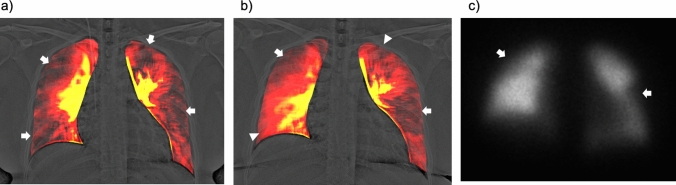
Dynamic chest radiography (DCR)-based detection of acute pulmonary thromboembolism (PTE) progression to chronic thromboembolic pulmonary hypertension (CTEPH) in a 32-year-old woman. In the acute PTE phase, DCR demonstrated multiple perfusion defects, suggesting pulmonary embolism in both lungs (a, arrows). Even after 6 months of anticoagulation therapy, the patient experienced mild dyspnea. Repeat DCR demonstrates improved perfusion defects (b, arrowheads) and persistent large perfusion defects in the bilateral lung fields (b, arrows), which are very similar to the findings of subsequent ventilation/perfusion (V/Q) scintigraphy (V/Q mismatch; c, arrows). Pulmonary hypertension was confirmed using invasive right heart catheterization, and a diagnosis of CTEPH was confirmed. *Reproduced from Ref 29 (Yamasaki Y, et al. Chronic thromboembolic pulmonary hypertension after acute pulmonary thromboembolism revealed by dynamic chest radiography. Eur Heart J Cardiovasc Imaging. 2022;23(6):e264-e265), which is an open-access article distributed under the Creative Commons Attribution License
